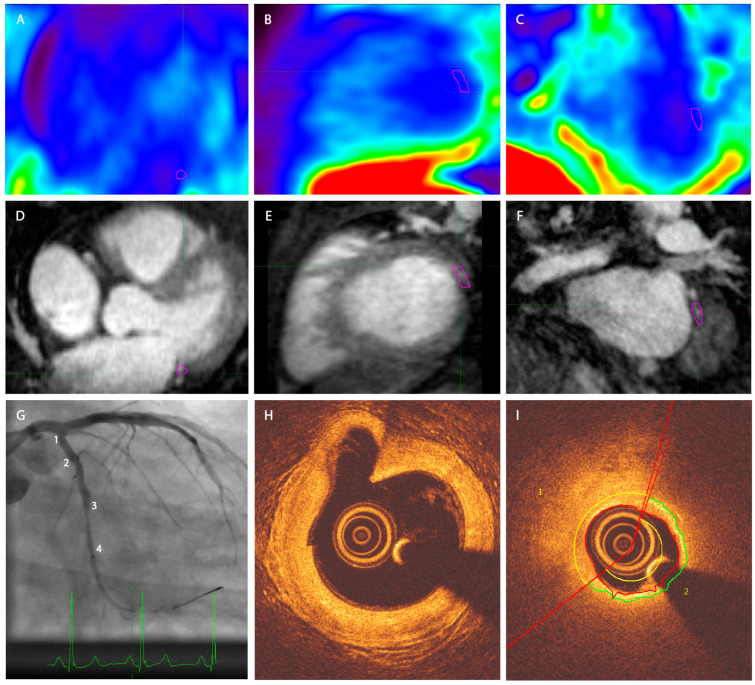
Exemplary PET-MRI and OCT image of one patient. (A–C) Axial, sagittal, and coronal PET. (D–F) Axial, sagittal, and coronal MRI, (G) angiography. Numbers 1–4 represent four different angiographical landmarks to correlate with OCT. A significant stenosis is present between landmarks 3 and 4. (H) OCT image corresponding to landmark 1 in panel (G), a large bifurcating vessel can be distinguished in the left upper quadrant of the image; (I) OCT image in the region of the stenosis as displayed in panel (G). The vessel segment between 2 and 3 contains a plaque with a thin cap on the OCT analysis. The purple VOI in panels (A–F) delineates the target plaque in the left circumflex artery of this patient. No increased tracer uptake was visually detectable in the coronary arteries.
